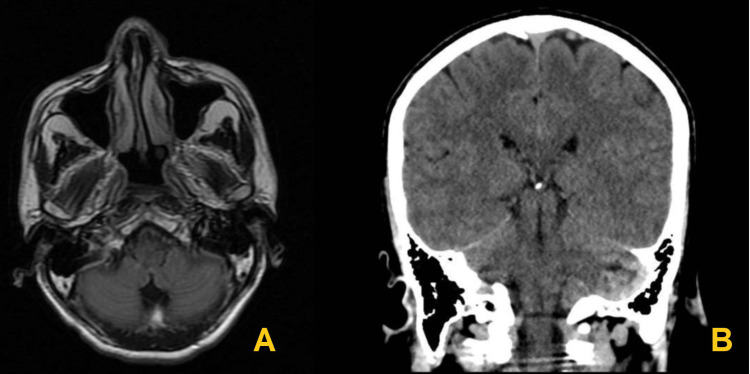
Head imaging. (A) MRI of the head in T2 FLAIR shows the output of cranial nerves IX and X without any alterations. (B) A simple tomography of the skull in the sagittal section, without structural alterations that compromise the anatomy of the head or trunk. MRI, magnetic resonance imaging; FLAIR, fluid-attenuated inversion recovery
